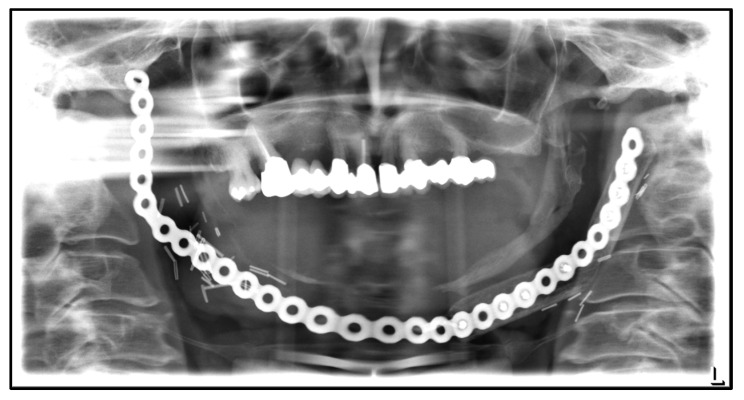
Dental X-ray after removal of the osteolytic destructed lateral right and anterior fibula segment and following re-osteosynthesis due to chronic inflammation.
